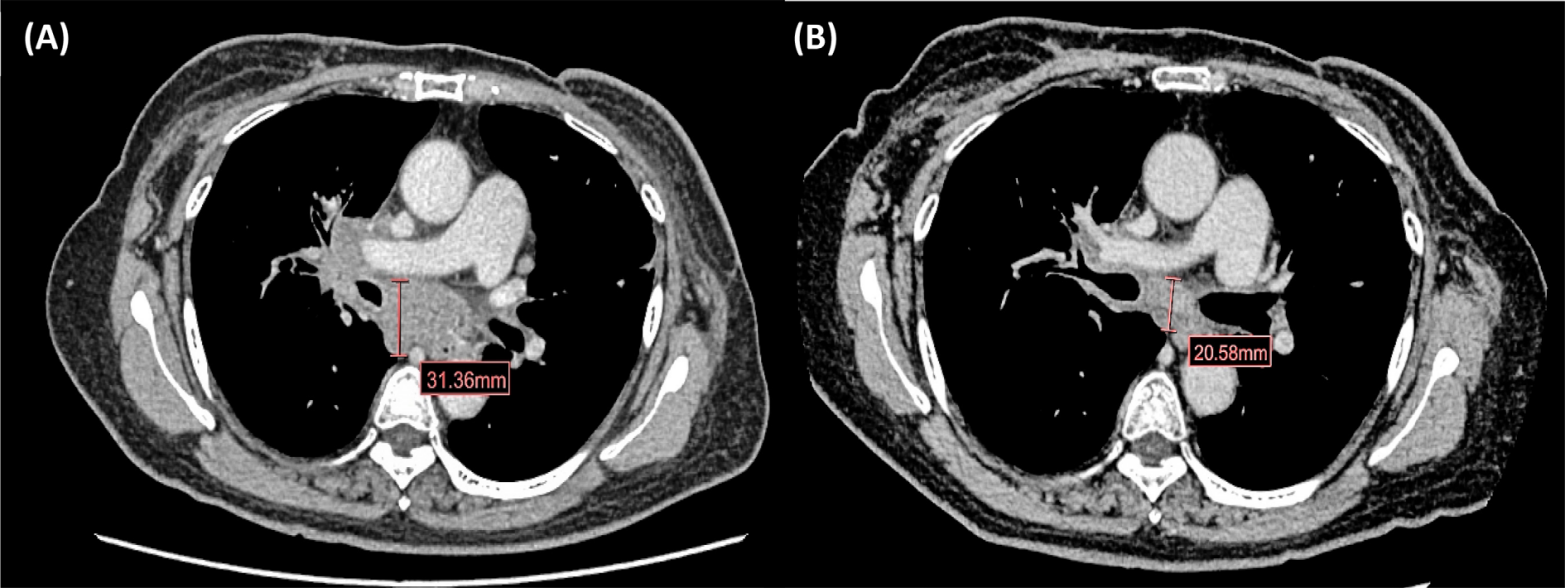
Chest computed tomography (CT) scan of target lesion during palbociclib plus fulvestrant. In April 2019, baseline chest CT scan before fulvestrant plus palbociclib was started (A); In September 2019, chest CT scan showed decreasing in size of mediastinal and hilar lymph nodes (chest CT scan before changing palbociclib to ribociclib plus fulvestrant according to Thai OCPA) (B)
